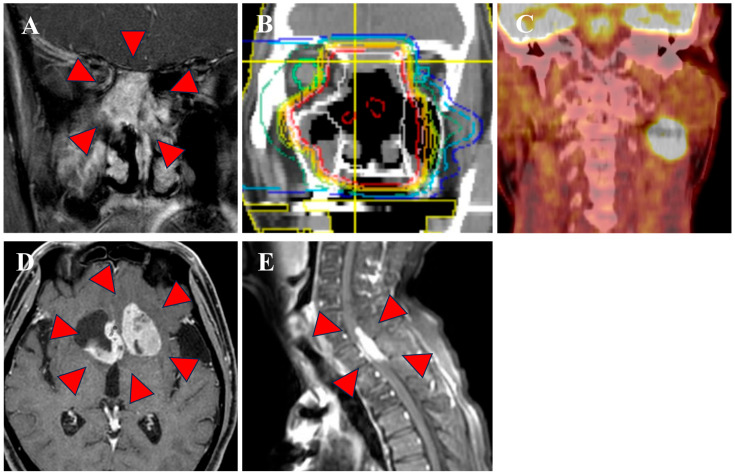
Case of a 72-year old man with SNEC in the right nasal cavity (Case 2). (A) Pre-treatment MRI; (B) PBT plan; (C) Regional recurrence; (D) Brain metastasis; (E) Intraspinal metastasis; Red triangles indicate primary or metastatic lesions.
